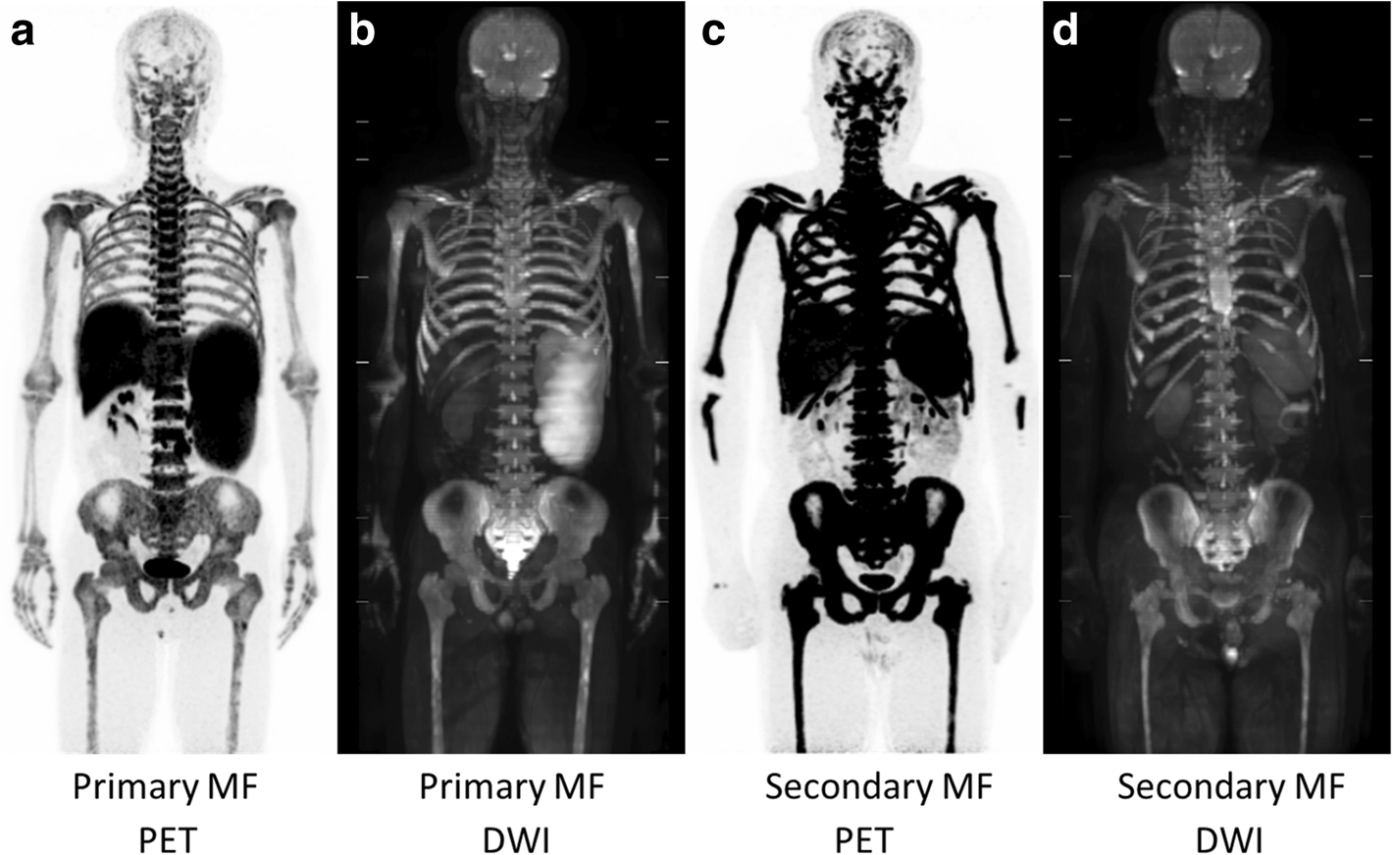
Maximum intensity projection ^18^F-FLT PET (a, c) and DWI (b, d) images of MF patients. A primary MF patient exhibited normal or slightly decreased bone marrow activity in the central skeleton, but they had marked expansion to the distal extremities and high uptake of the tracer in the enlarged spleen (a). On the other hand, a secondary (post-ET) MF patient had marked ^18^F-FLT uptake in bone marrow (c). Whole-body DWI displayed homogenously high signal intensities in bone marrow of primary and secondary MF patients (b, d, respectively)
